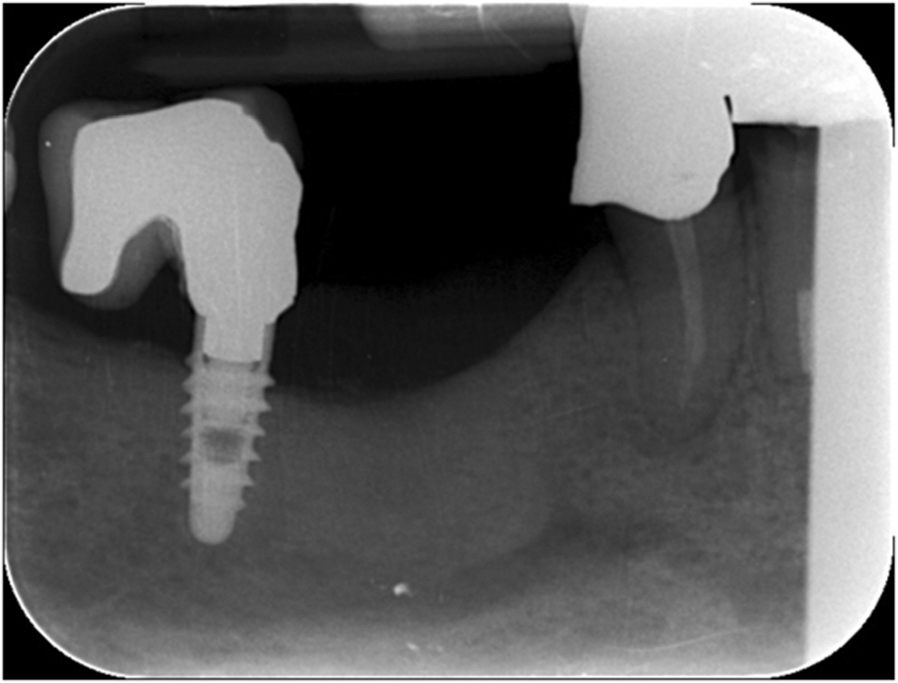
Intraoral radiography: regenerated bone after 5 months
